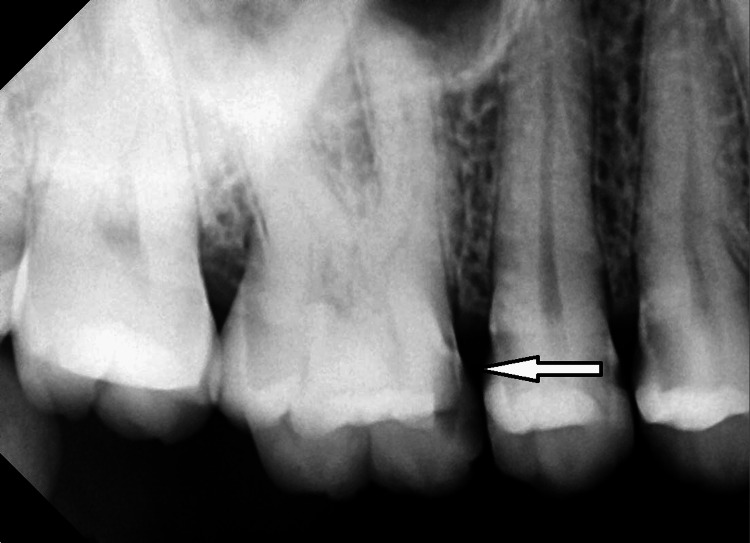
Radiographic image preoperative
